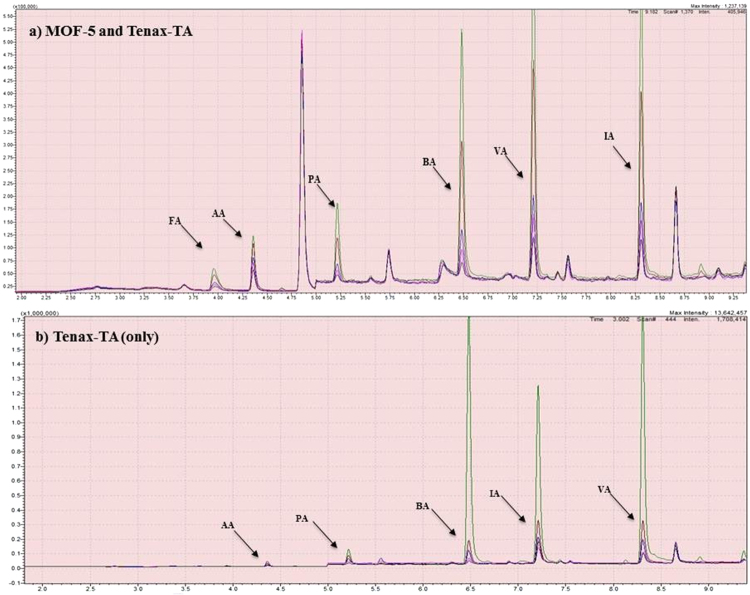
Chromatograms of a mixture of carbonyl compounds used as the L-WS (analytical volume = 1 µL) using MOF-5 + Tenax-TA as ST and CT sorbents. Colors represent different masses as follows: green = 95 to 98 ng, brown = 47 to 49 ng, blue = 23 to 24 ng, pink = 10 ng, black = 4.8 ng.
